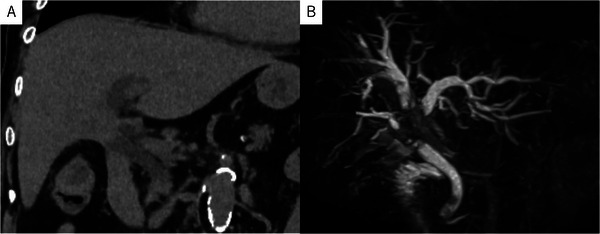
Non‐contrast CT showing wall thickening of the hilar bile duct and dilatation of the intrahepatic bile ducts. MRCP demonstrating dilated intrahepatic bile duct−right anterior, right posterior, and left hepatic ducts−with abrupt obstruction at the hepatic hilum. CT: computed tomography, MRCP: magnetic resonance cholangiopancreatography.
